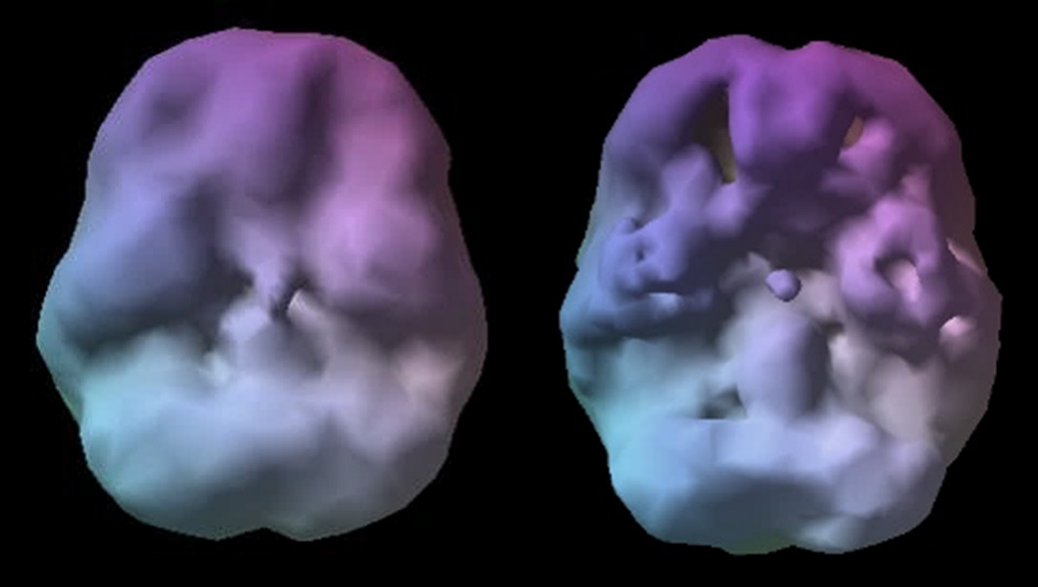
Healthy SPECT image compared to C's pre-treatment SPECT image. A healthy SPECT image (L) shows full, even, symmetrical activity compared to C's pre-treatment SPECT image (R) with low activity, especially in the prefrontal cortex and temporal lobes. Photon emission was captured using a high-resolution Picker (Phillips) Prism 3,000 triple-headed gamma camera with fan beam collimator with data collected in 128 × 128 matrices, yielding 120 images per scan with each image separated by three degrees spanning 360 degrees. A low pass filter was applied with a high cutoff. A Chang attenuation correction was performed using linear methods. All images were processed using Odyssey software (Picker), with transaxial slices oriented horizontal to the AC-PC line. Coronal, sagittal, and transaxial slice images (6.6 mm apart, unsmoothed) were then rendered in the Odyssey step-20 scale. Image acquisition and processing methods apply to all images presented in this article.
